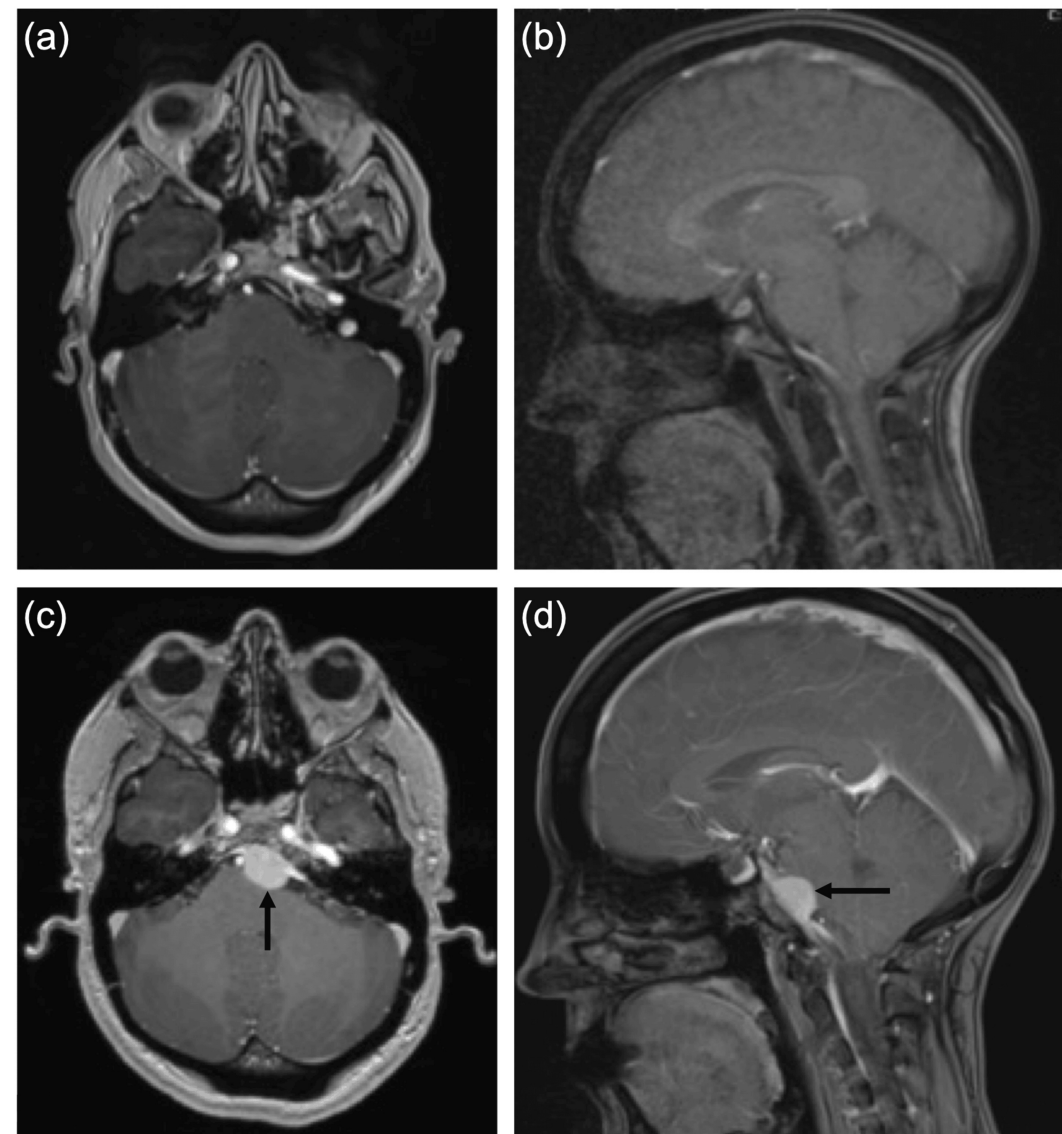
Recurrence of meningioma (a-b) 5-year magnetic resonance imaging (MRI) following gamma knife radiosurgery shows stable appearance of a left clival meningioma. (c-d) Recurrence of clival left central meningioma 11 years after initial diagnosis. Tumour measures 15 mm in depth, displacing the basilar artery to the right and indents the anterior pons without oedema. No extension into the Meckel’s cave or left internal auditory meatus noted.
